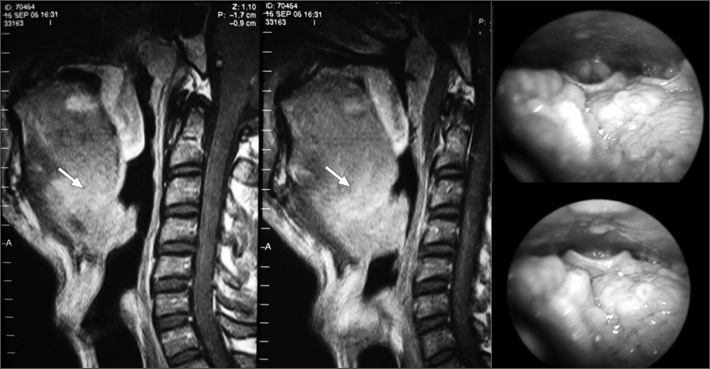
Lingual tonsil hyperplasia - Laryngoscopic and magnetic resonance images demonstrating lingual tonsil hyperplasia.
